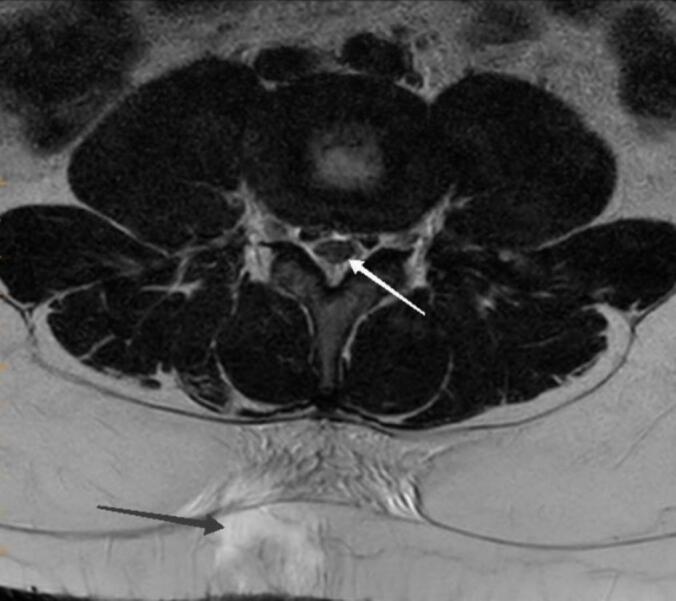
Axial T2-weighted lumbar MRI image, white arrow show distortion and clumping of nerve roots at the level of injury, according to spinal bifida occulta in lumbosacral levels. Black arrow show CSF effusion.
